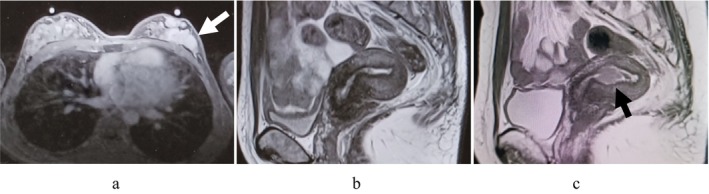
(a) Breast MRI image (T1‐weighted transverse view). 38 × 31 × 18 mm mass with irregular margins is seen in the C region of the left breast (white arrow). (b) Pelvic MRI scan showed a lesion in the uterine lumen with mixed mild low and high signal on T2‐weighted images. (c) Pelvic MRI scan shows an 18‐mm mass lesion in the uterine lumen with mild high signal on a well demarcated T2‐weighted image (black arrow).
